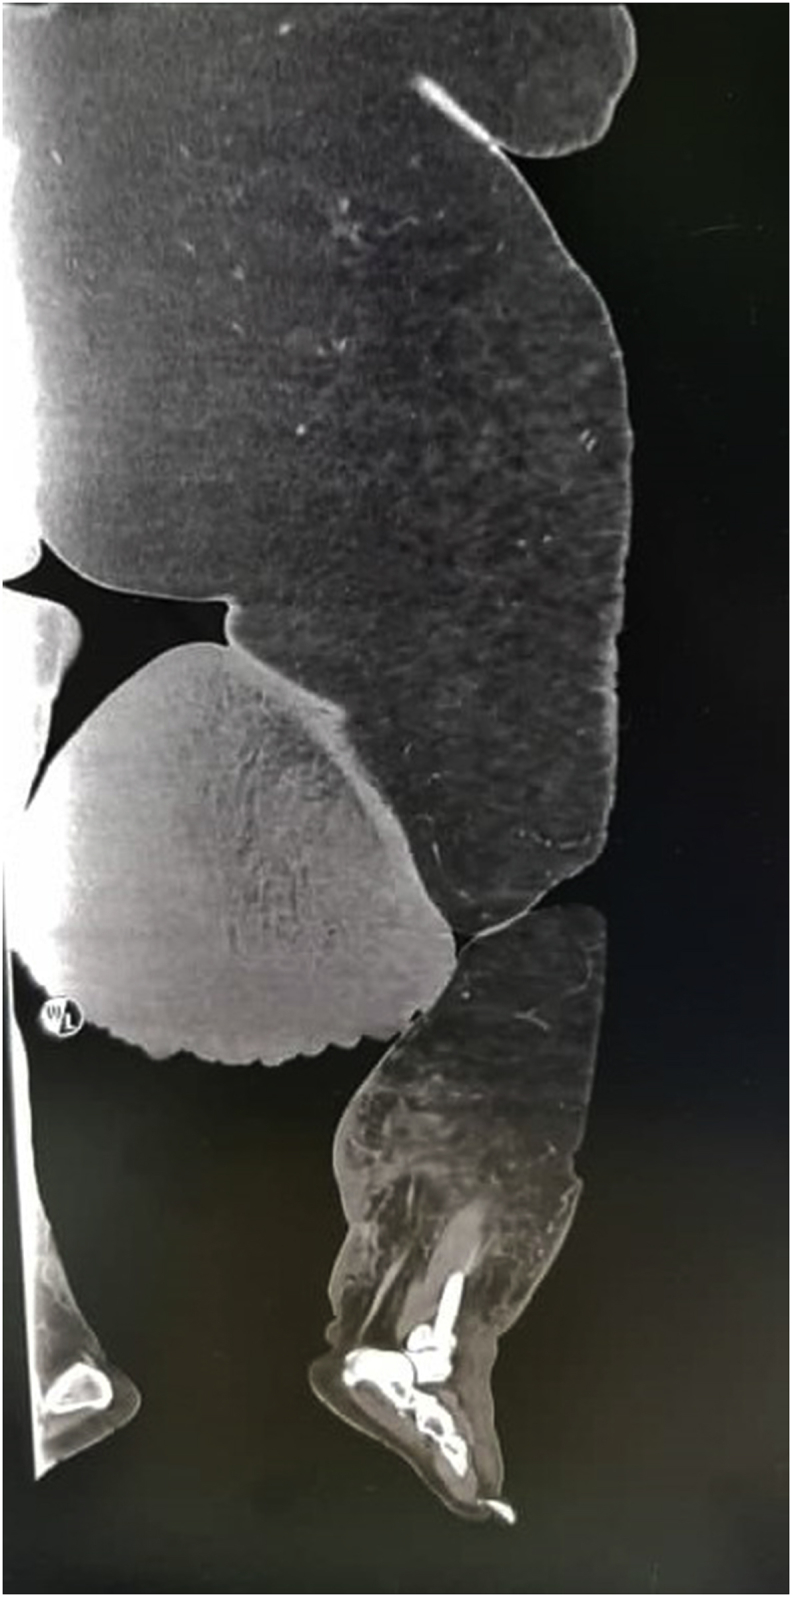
Coronal view of CTA of the left lower limb showing a large hyperdense pedunculated soft tissue mass arising from the medial compartment of the proximal left thigh.
